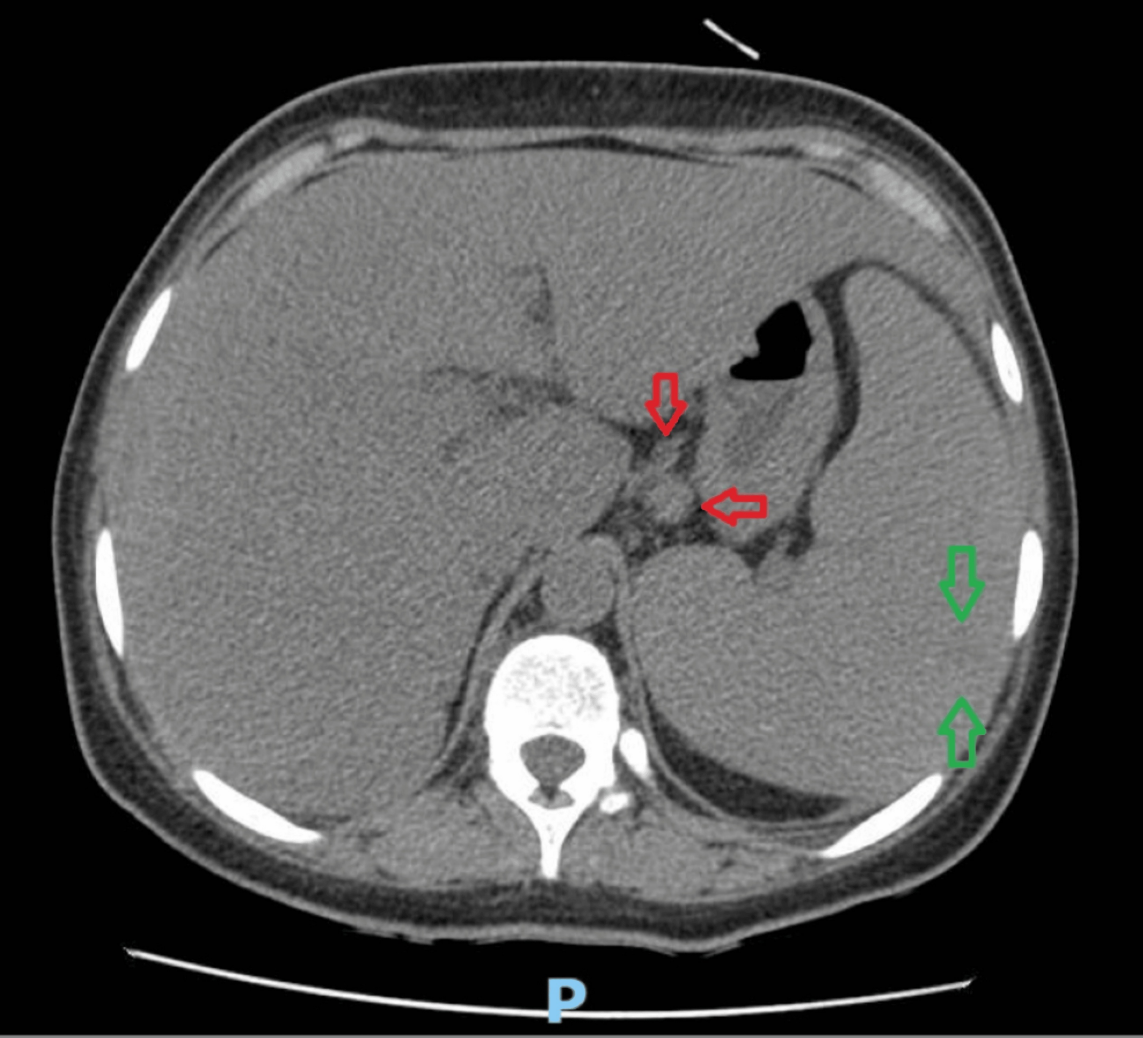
Axial contrast-enhanced CT scan of the upper abdomen (arterial phase) revealing multiple enlarged retroperitoneal, mesenteric, and iliac lymph nodes (red arrows), in addition to splenomegaly with probable infarcted areas (green arrows) characterized by cuneiform hypervascular patterns CT: computed tomography
